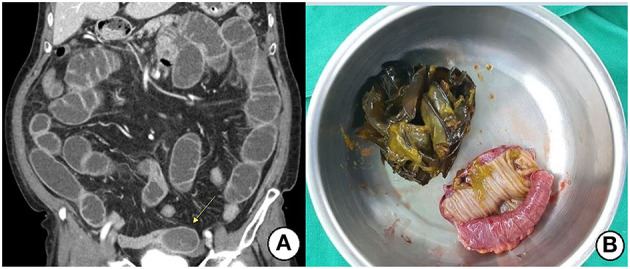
(A) Contrast-enhanced CT shows an ovoid heterogeneous mass (arrow) and proximal distended small bowel. (B) Segmental resection and anastomosis were performed due to the large-sized seaweeds bolus.
